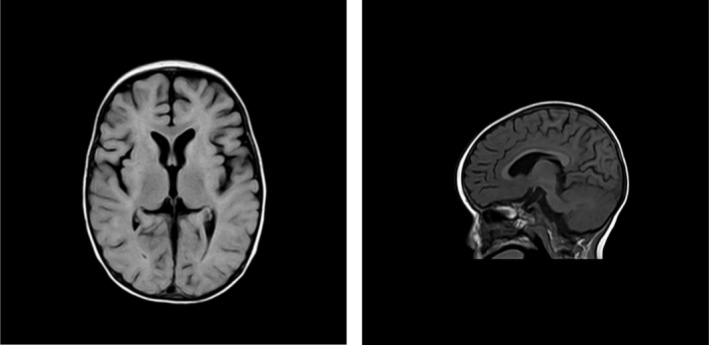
FIGURE 1 Brain MRI performed at 13 months of age. The examination showed deepened cerebral sulci suggesting cerebral atrophy, enlarged ventricular system, dysgenesis of the corpus callosum, and age‐appropriate myelination.
